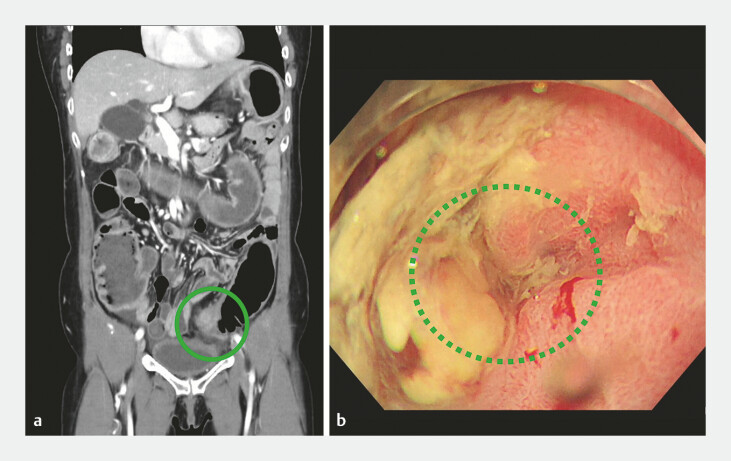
Computed tomography (CT) and endoscopic image of sigmoid colon cancer.
